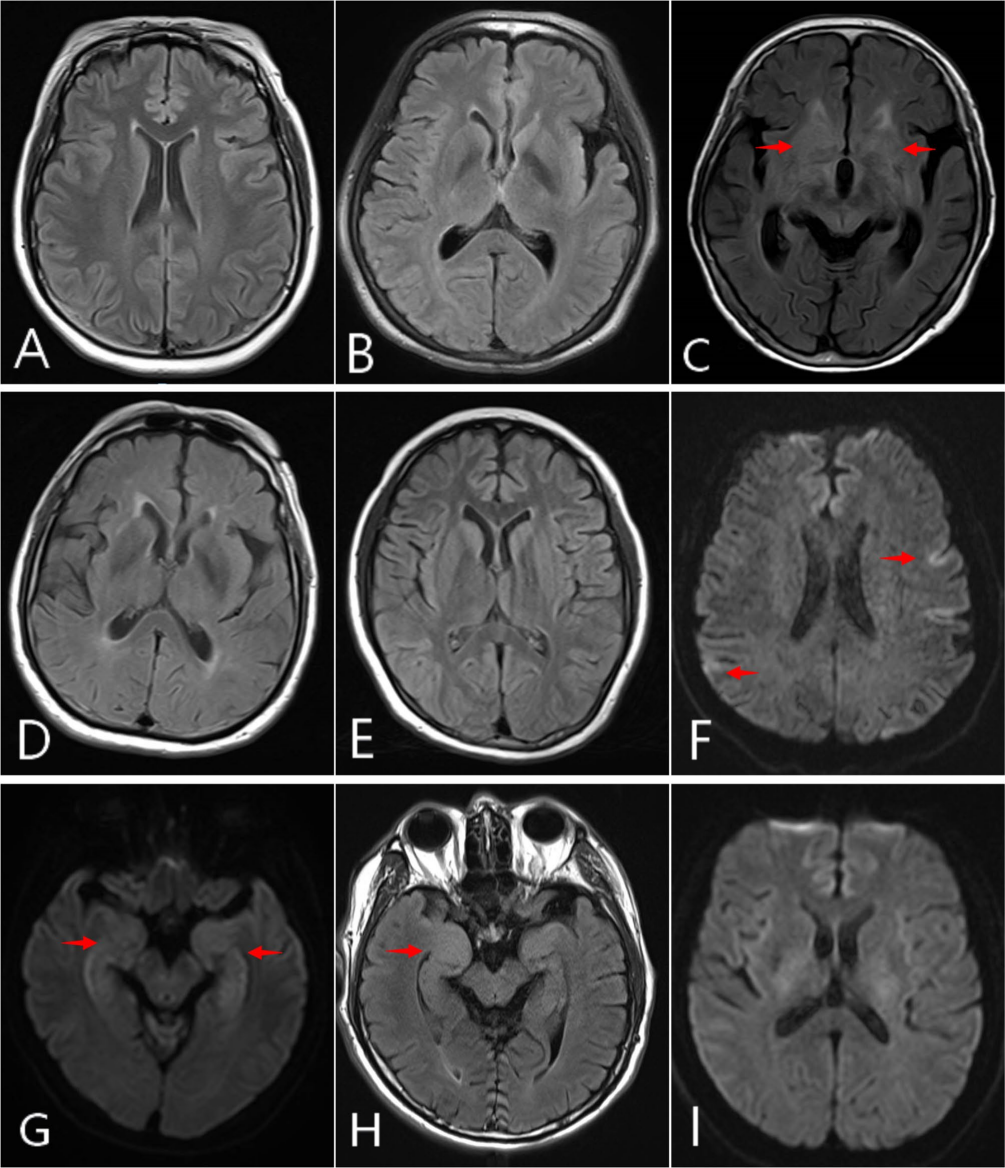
Cerebral MRI changes of 9 cases. Cerebral MRI revealed no abnormalities (A in patient 1; B in patient 2; D in patient 4; E in patient 5; I in patient 9). T2/Flair showed hyperintensities mostly involving the temporal lobe, parietal lobe, and basal ganglia area in patient 3 (C). Diffusion weighted imaging (DWI) showed hyperintensities involving the temporal and occipital cortex in patient 6 (F); DWI showed symmetrical hyperintensities in the bilateral temporal and insular cortex in patient 7 (G); and T2/flair showed swelling of the right hippocampus in patient 8 (H)
